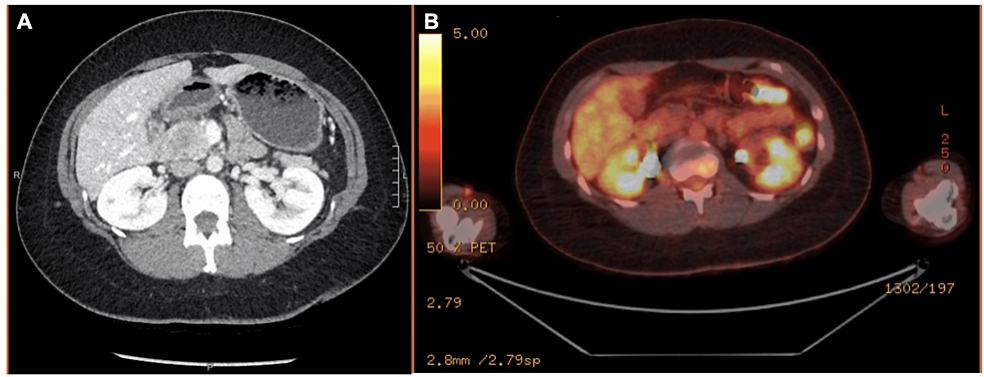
Cross-sectional imaging revealing new pancreatic head mass suspicious for recurrence 1.5 years after wide local resection of left upper thigh mass as noted on (A) CT and (B) FDG-PET. CT, computed tomography; FDG-PET, fluorodeoxyglucose positron emission tomography
